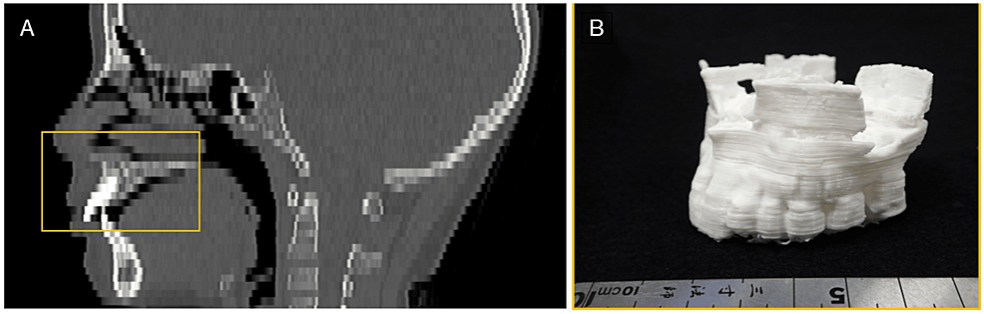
An example of 3D modeling from DICOM image data with too-thick slices. An example of a maxilla (square) that had to be segmented and 3D printed, even though the quality of the original images for STL model creation was poor. (A) CT median sagittal image of the head. A thick slice thickness results in stepped edges and poor reproduction of detail. (B) 3D model fabricated from these images. 3D CAD software was used to edit polygons, including smoothing the STL model, but there were limitations, resulting in a rough 3D model. CAD: Computer-aided design, STL: Stereolithography, DICOM: Digital Imaging and Communications in Medicine.
